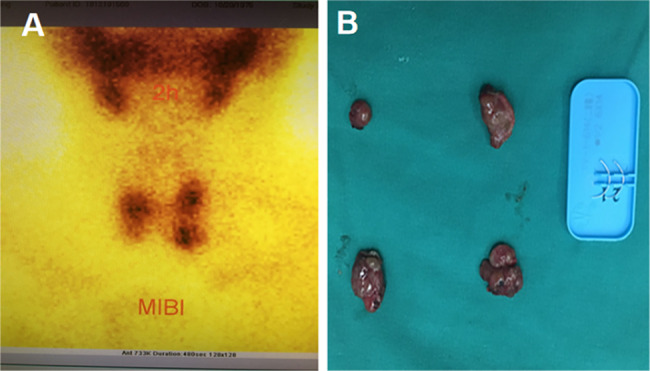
Parathyroid status of the patient. (A) Preoperative parathyroid scintigraphy.Shows four hyperfunctioning parathyroid glands, consistent with secondary hyperparathyroidism. (B) Intraoperative view of excised parathyroid glands.Displays all four parathyroid glands that were excised
